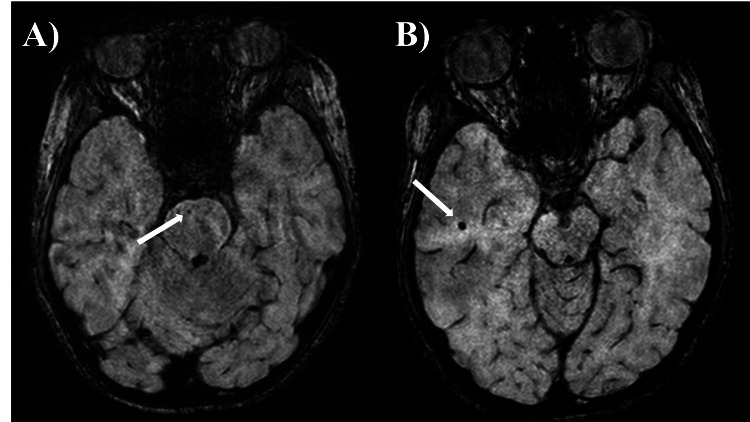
Brain magnetic resonance imaging at the 11 and 13 years of age The appearance of new lesions in the pons and right temporal lobe was confirmed on susceptibility-weighted imaging.
